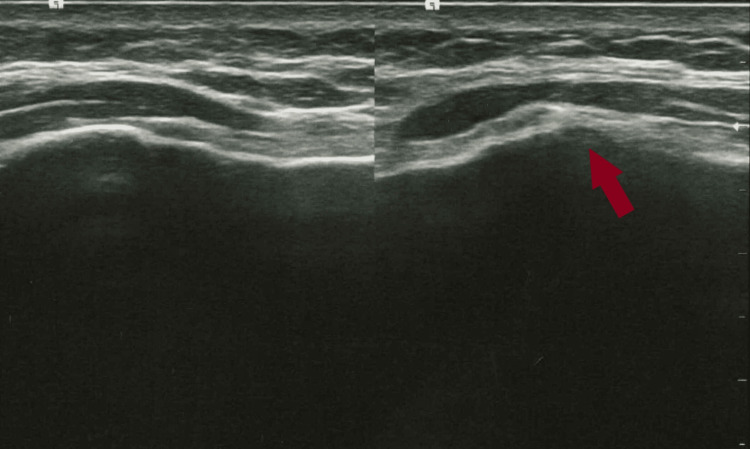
The Image of an ultrasound scan of the sternocostal joints at the follow-up visit
